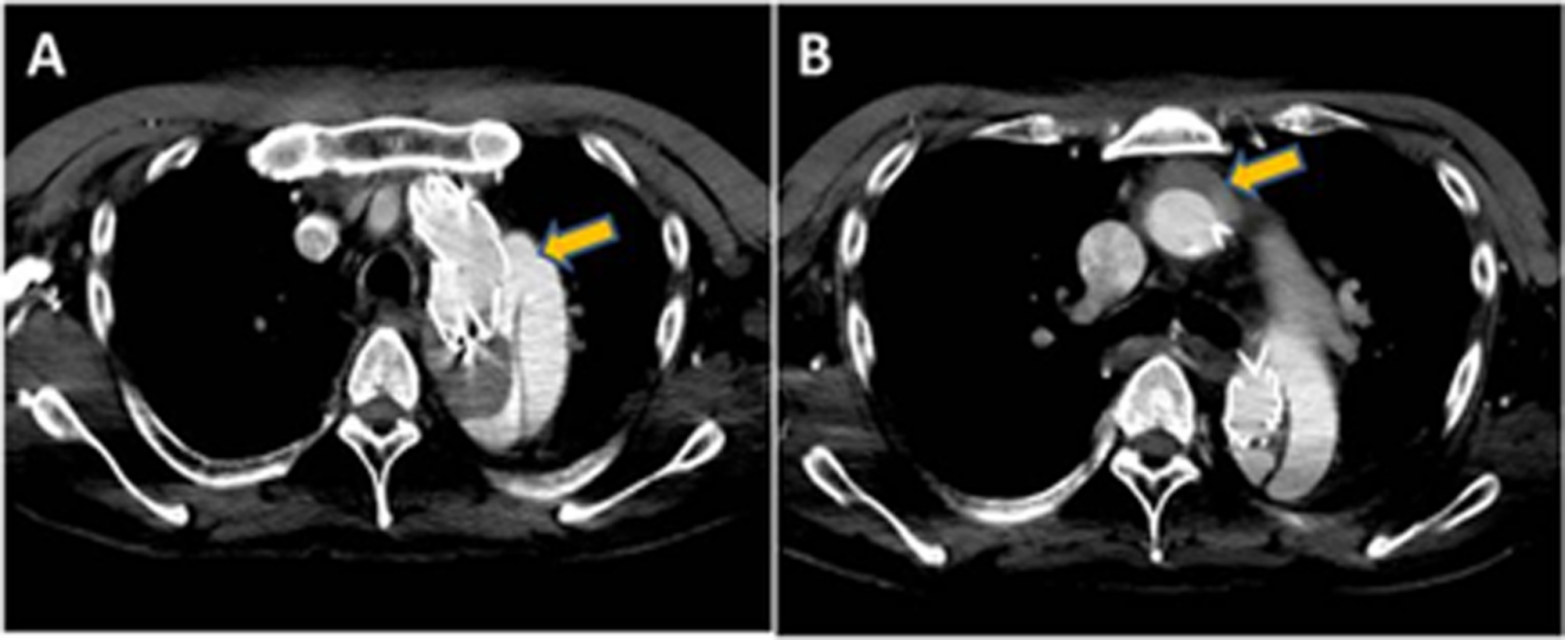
Computed tomography findings: The enlarged patent false lumen of the descending aorta with a primary entry (A) and thrombosis of the ascending aorta (B)
